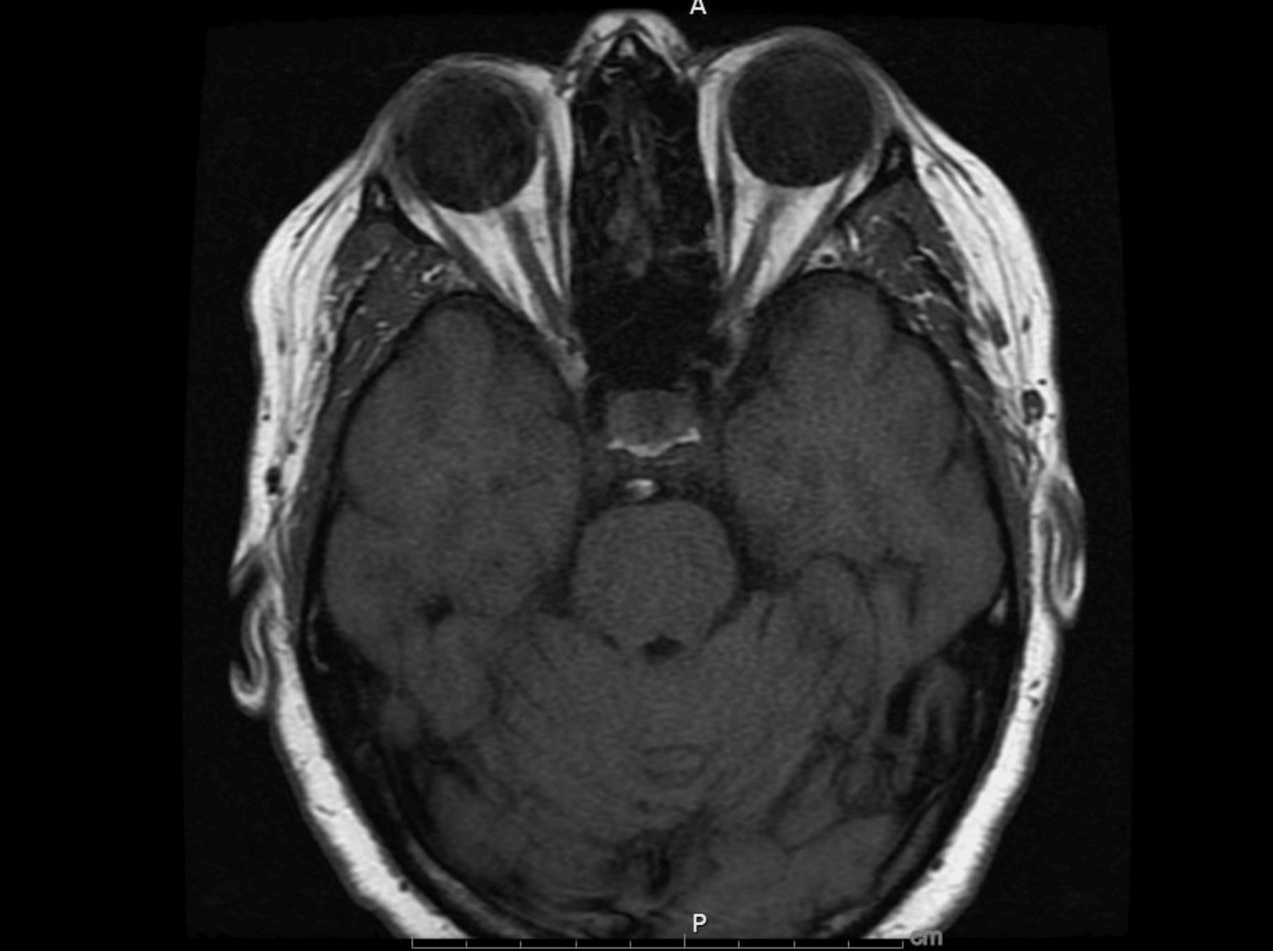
Magnetic resonance imaging (MRI) brain and orbits T1 weighted showing normal anatomy of orbits and retro-orbital structures
